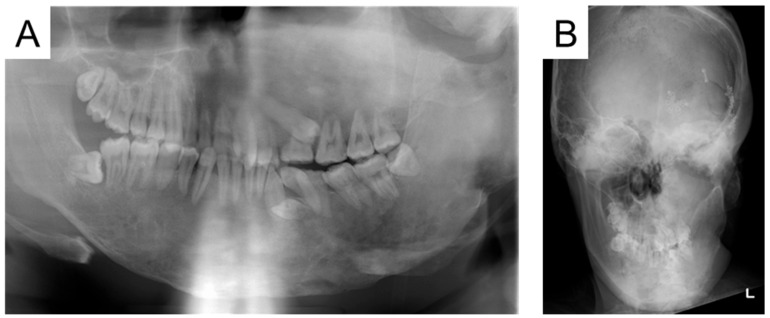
Radiographic examination at the age of 27 years and 10 months ((A): Panoramic photo-graph, (B): Antero-posterior-view skull).
